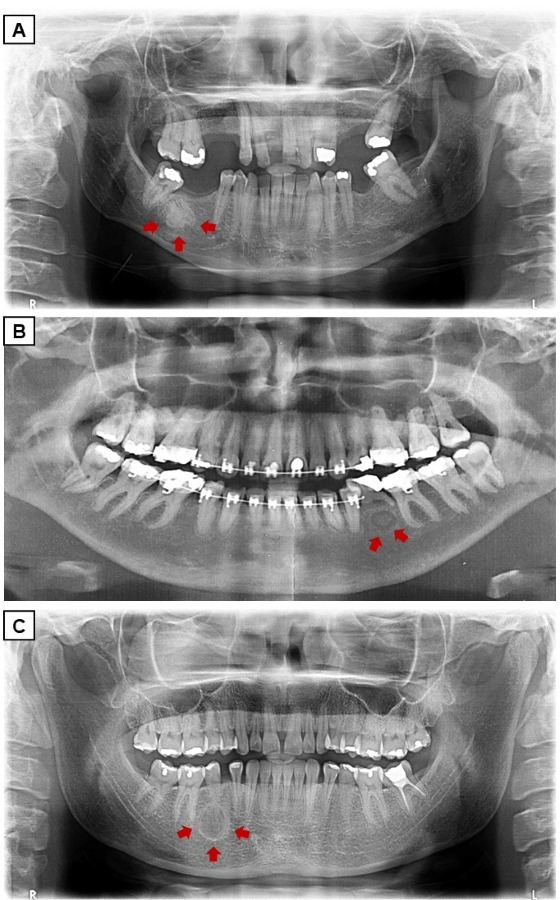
Radiographic characteristics of benign bone-forming tumors of the jaws on panoramic radiographs. (A) Osteoma exhibiting a homogeneous, well-defined radiopaque lesion, located in the edentulous region of the right mandibular body corresponding to the region of tooth 47. (B) Osteoid osteoma presenting as a well-defined radiopaque lesion, surrounded by a radiolucent halo, located in the periapical region of tooth 75 (the patient had agenesis of tooth 35). (C) Osteoblastoma showing a well-defined radiolucent lesion, surrounded by a radiopaque halo, located in the periapical region of tooth 45 in the right mandibular body.
